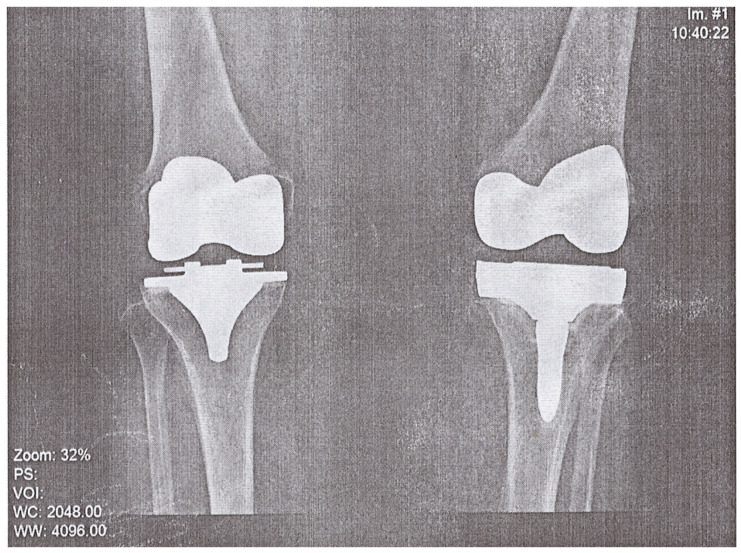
AP radiography, pre-operative, of suspected left knee PJI.
